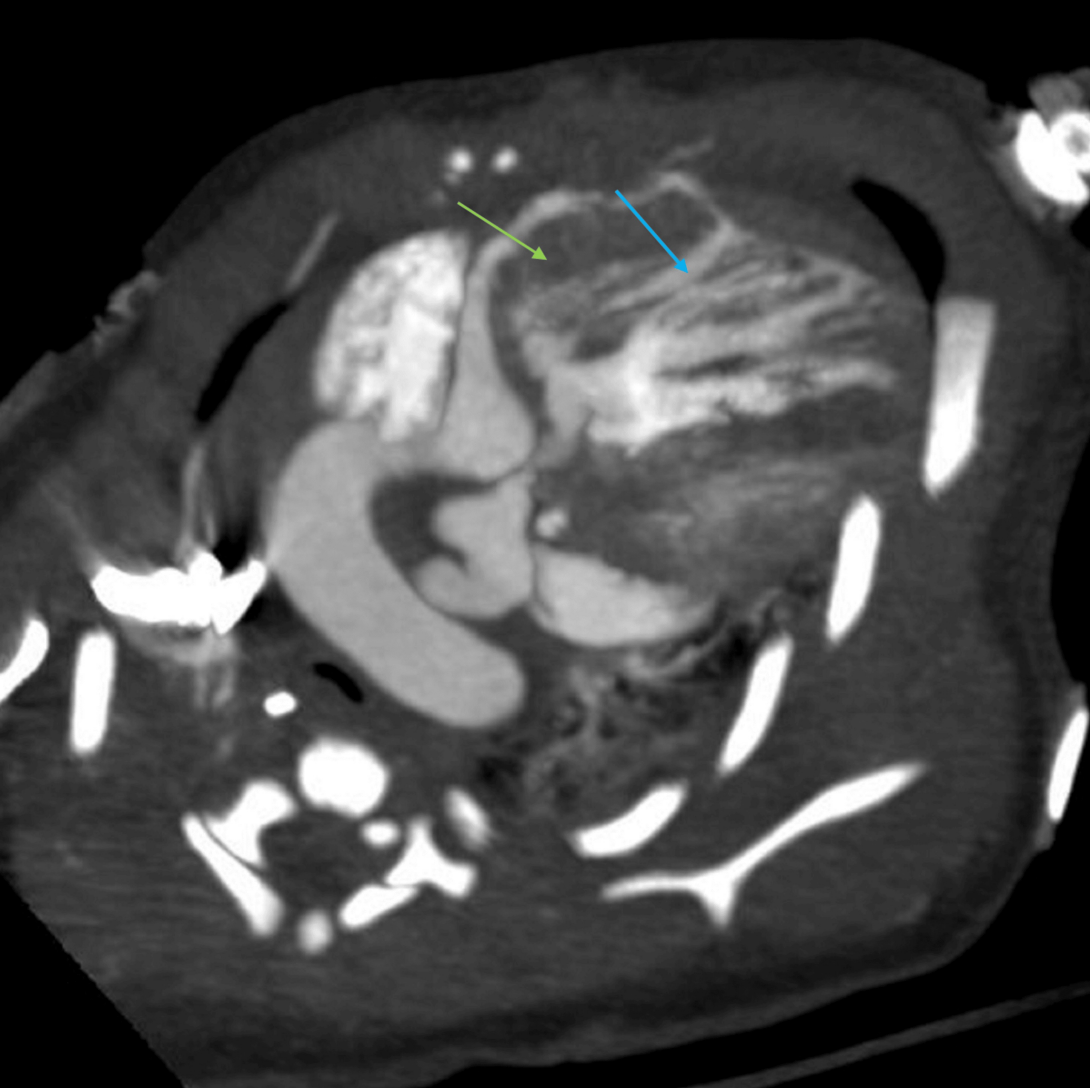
Axial computed tomography image of the markedly dilated coronary arteries (blue arrow) communicating with the right ventricle (green arrow).
